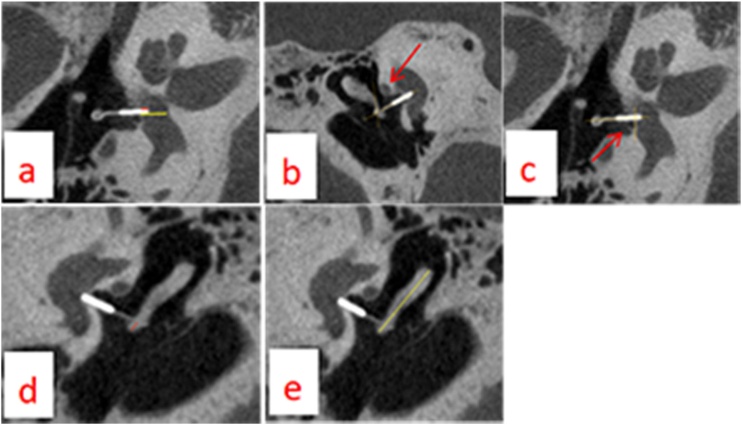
Images of the U-HRCT from OS patients undergone stapes surgery. Measurement of stapes prothesis position from (a to c). (a) The red line and yellow line represent absolute insertion depth and vestibular depth. The relative insertion depth is the ratio of both. (b) The two crossed yellow lines parallel to the long crus of incus and prothesis respectively, and red arrow shows the angle between them. (c) The two crossed yellow lines parallel to the prothesis and the footplate respectively, and red arrow shows the angle between them (d and e). The red line shows the distance between the hook and the end of incus, the yellow ratio of both line shows the length of incus. The relative distance is the.
